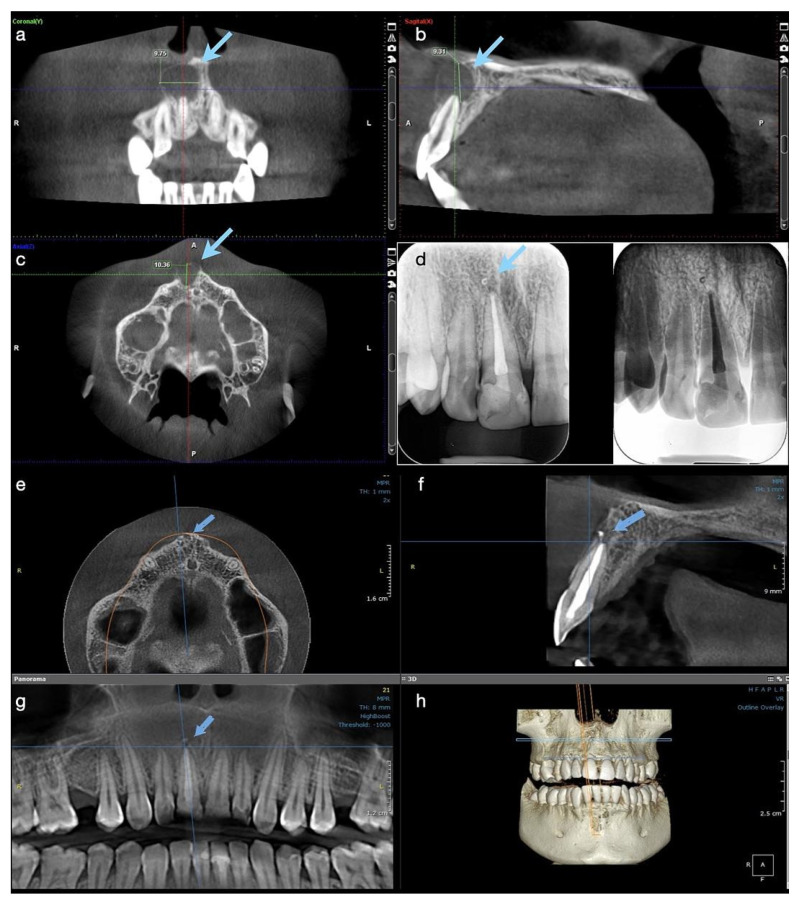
Case 1. Radiological investigations for tooth #11: (a–c) Initial CBCT scans with the measurements of the lesion’s size, indicated by blue arrows: mesiodistal diameter 9.75 mm, height 9.31 mm, buccolingual diameter 10.36 mm, with the interruption of the cortical buccal plate (9.75 × 9.31 × 10.36 mm); (d) control periapical X-ray at 6 months, showing the quality of the endodontic treatment and almost the complete healing of the lesion; (e–g) CBCT scans at 12 months showing the intact buccal cortical plate, the formation of new bone, and the almost complete healing of the lesion. A small enlargement of the periodontal ligament space is still observed apically, (h) 3D reconstruction where intact maxillary bone is observed.
